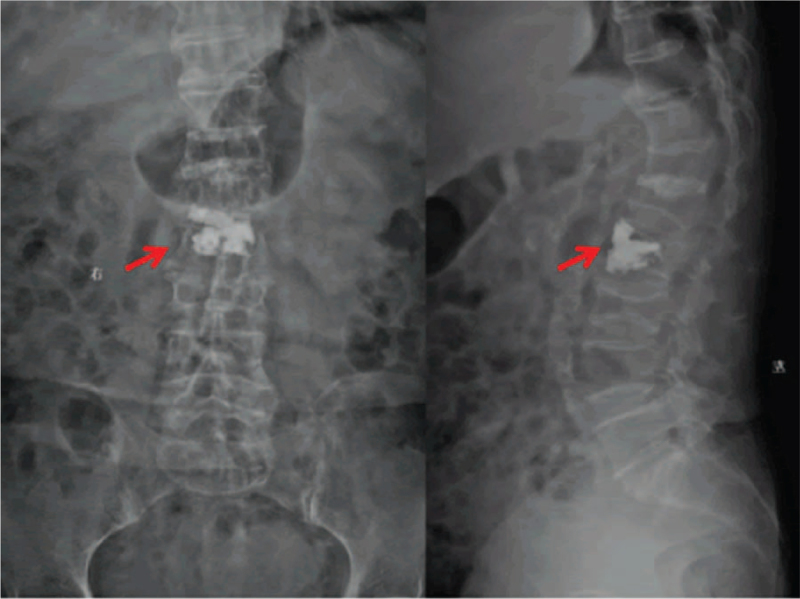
Postoperative radiographs showed that the L2 vertebral body was well filled with bone cement without intraspinal leakage (indicated by red arrow).
